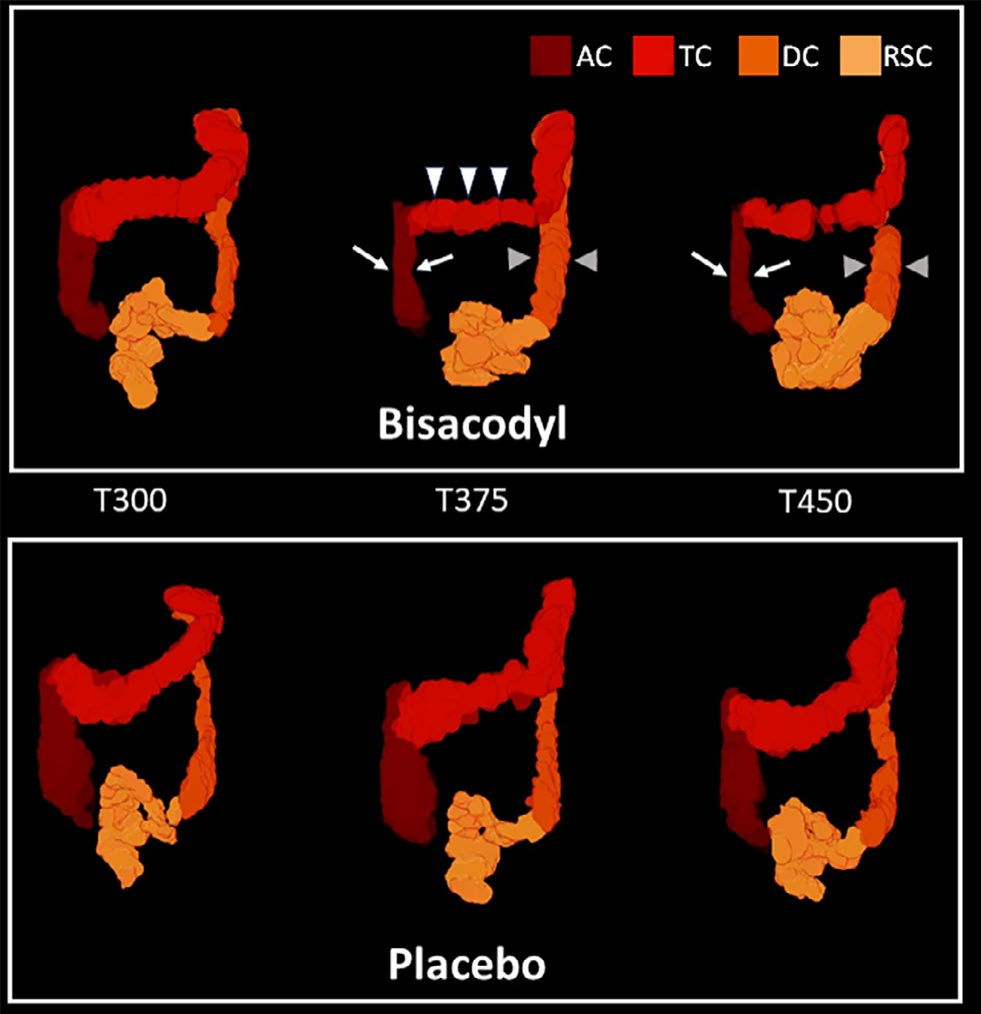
3D volume renderings of the MRI data, from a single participant in the repeated dose study. This shows the substantial changes in colon volumes after the third dose of bisacodyl when compared to placebo. The image at T 375 minutes shows the ascending colon volume reducing (contracting—white arrows) with simultaneous contraction of the transverse colon (white arrowheads). At the same time, the descending colon shows an increase in volume (relaxation/reduction in colon tone—light gray arrowheads). The rectosigmoid colon also shows an increase in volume (relaxation/reduction in colon tone) by 450 minutes. The volumes of the segments show little change over the same timeperiod in the placebo arm.
